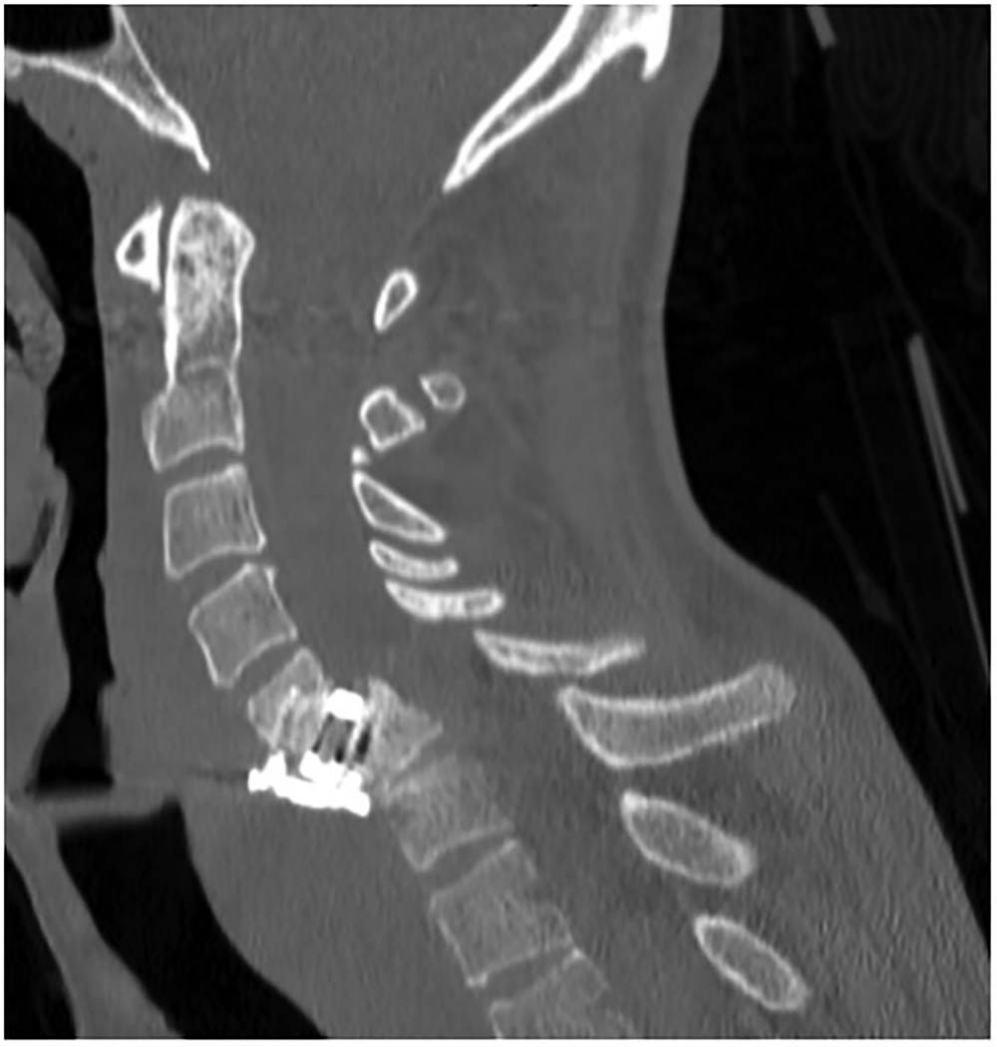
Sagittal CT of initial ACDF at cervical spine C5/C6 level over one year prior to esophageal perforation.
